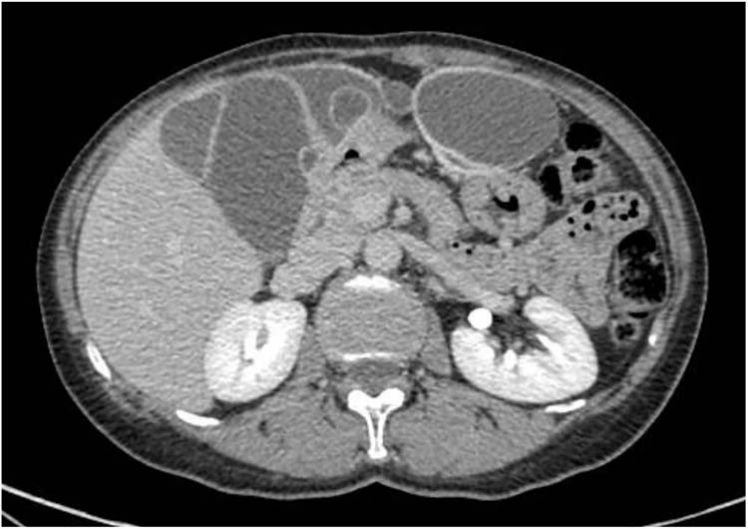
Axial CT-scan highlighting the abdominal pluriconcameral cystic formation.
